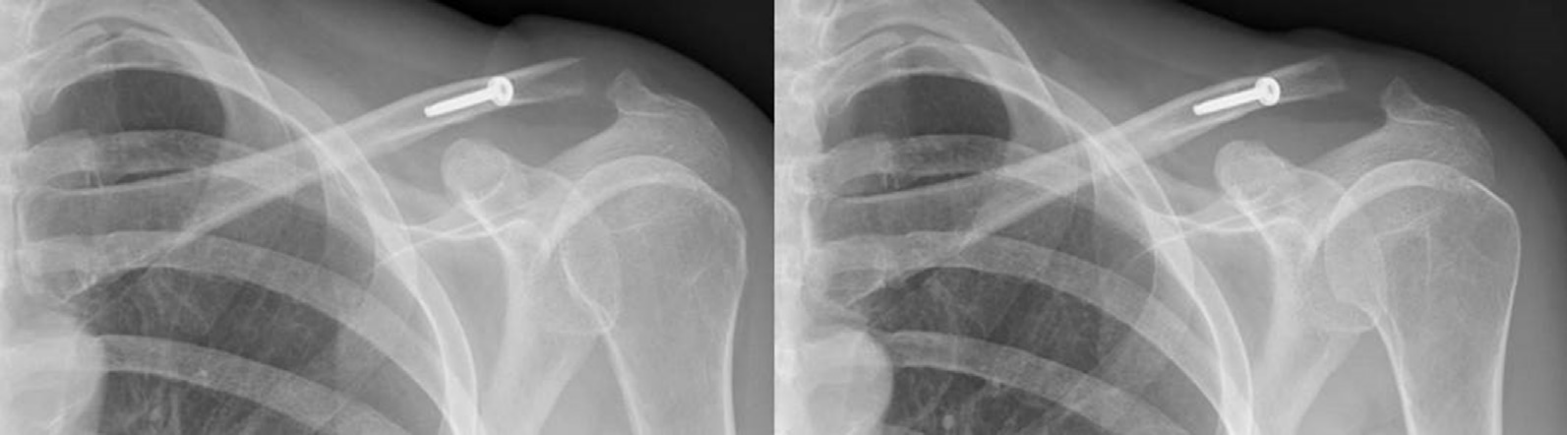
Radiographs at 6 weeks (left) and 3 months (right).
